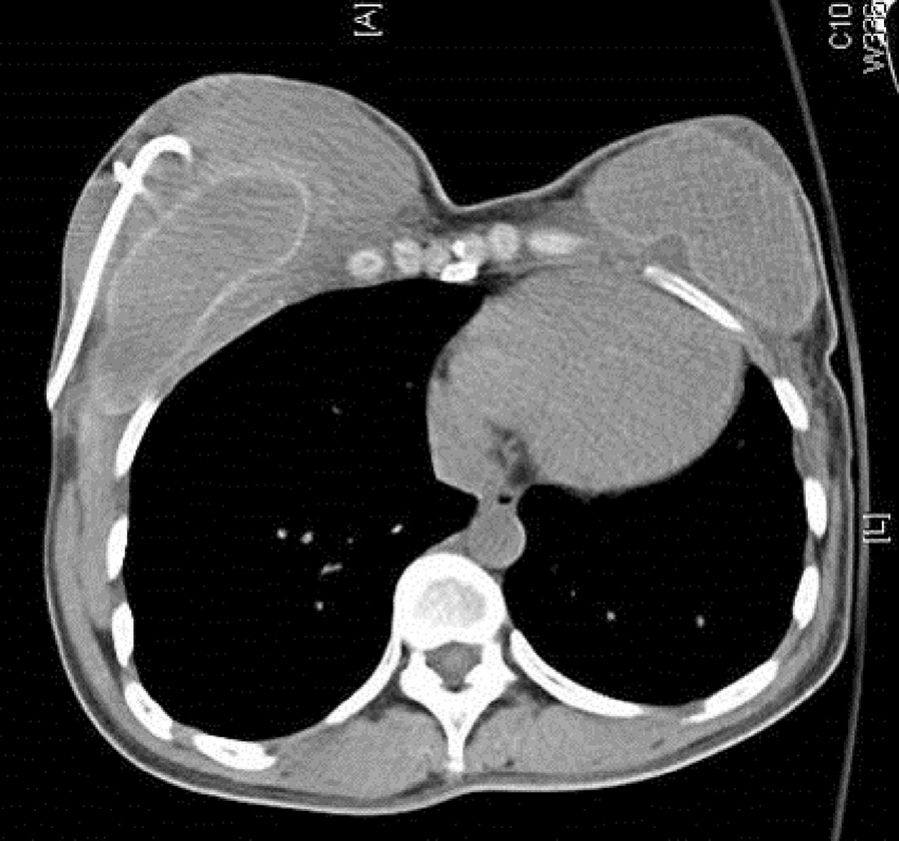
Computed tomography image of hematoma formation on the 14th day post simple mastectomy and immediate subpectoral silicon-implant reconstruction. Pigtail drainage tube was inserted and 250 ml hematoma was drained out
